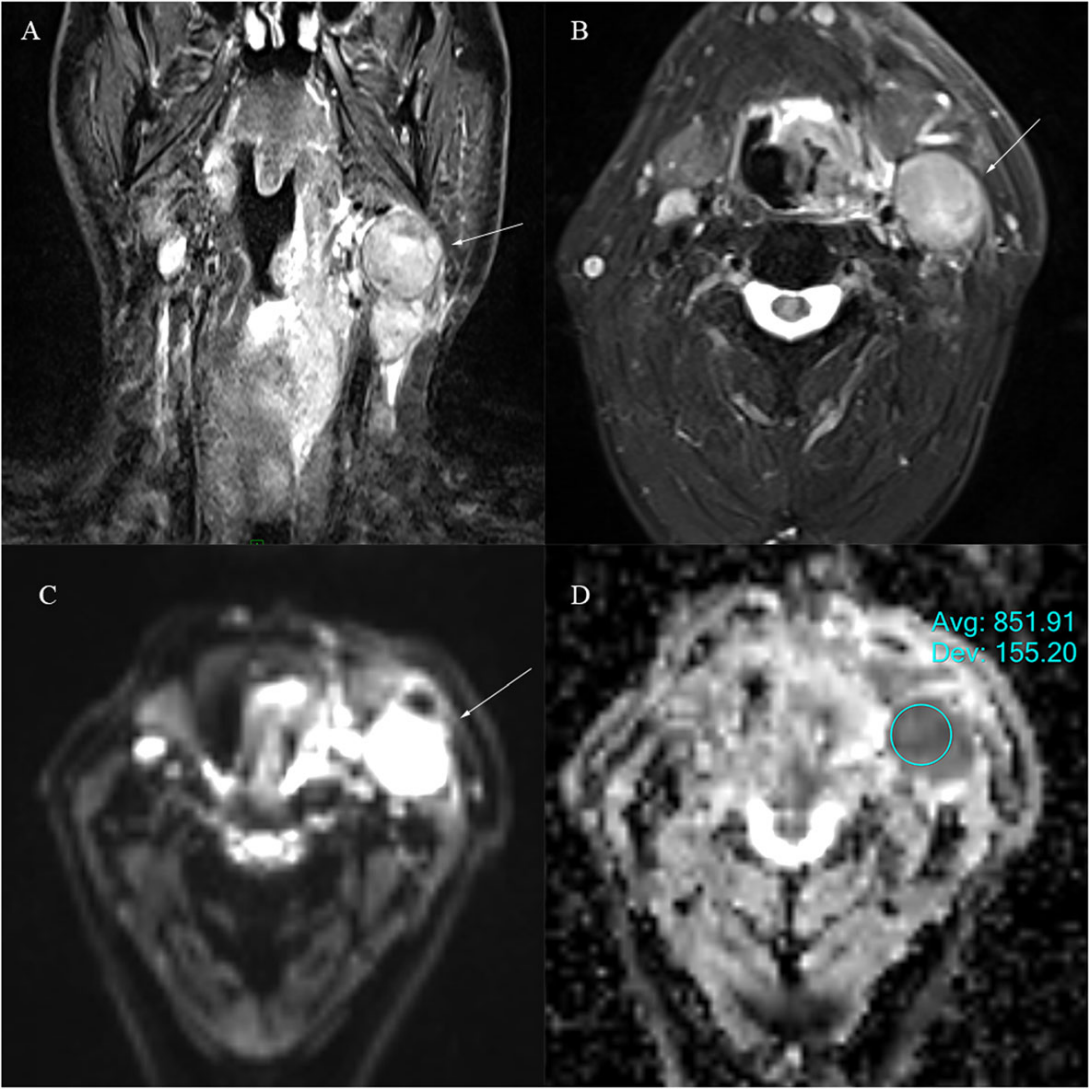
71 years old male with left positive para-pharyngeal enlarged lymph node from oropharyngeal SCC on the left side of the neck. a T2 coronal show enlarged lymph node axial dimensions, (34x35mm) (arrow). b T2 axial show the axial extension of the lymph node. c DWI show high signal at b value 800mm^2^/s and (d) the ADC map on the targeted lymph node show an ADC value of (0.851 ± 0.16*10^− 3^ mm^2^/s)
